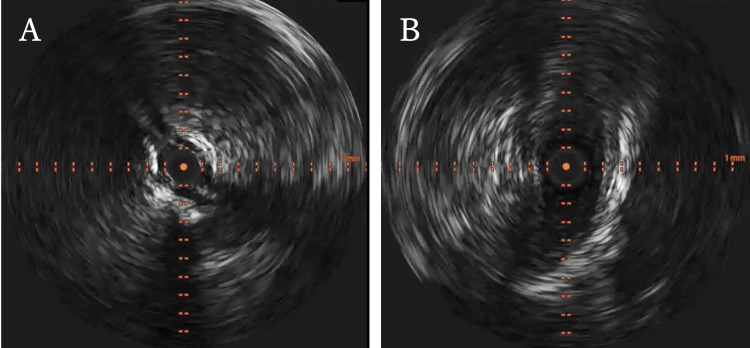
Intravascular ultrasound (IVUS) before and after treatment with Fogarty catheter. (A) IVUS revealed an iso-echoic lesion at the right popliteal artery. (B) Repeat IVUS revealed enlargement of the vascular lumen, with the lesion located outside the intima.
